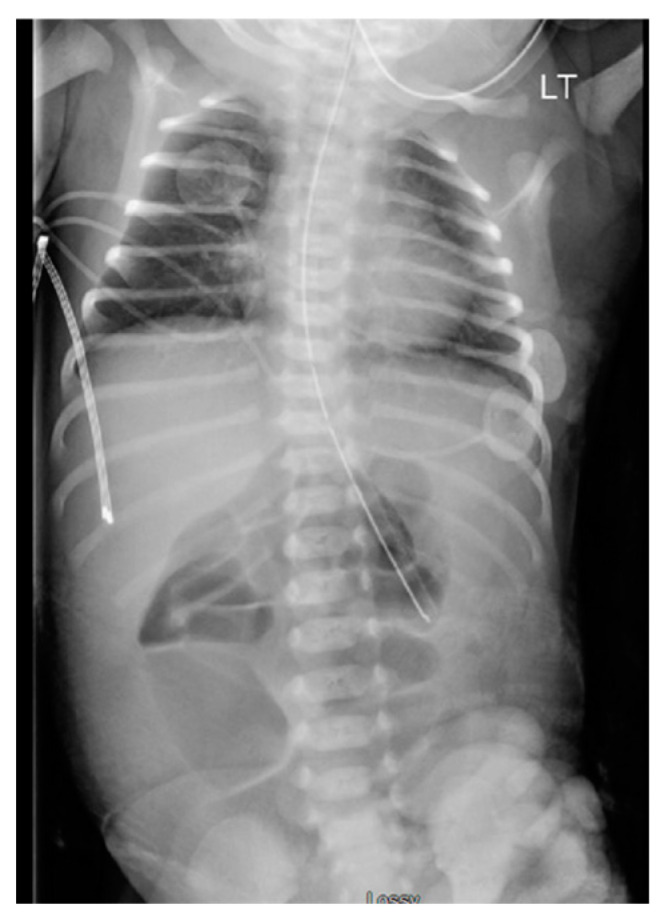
Day 1 abdominal X-ray revealing dilated intestines.
